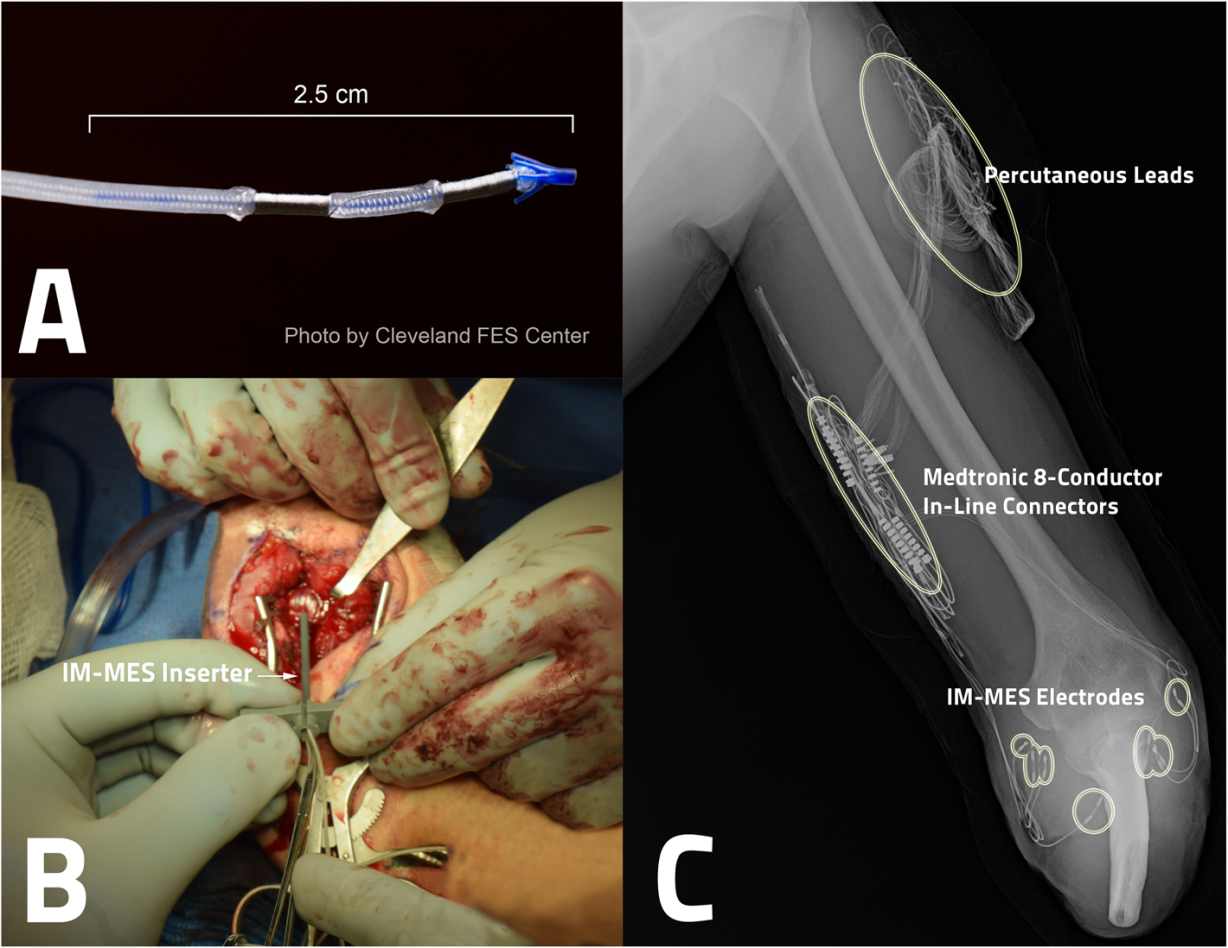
IM-MES Implantation. a One of the eight IM-MES electrodes. b One such electrode being surgically tunneled into a target muscle of the study participant’s residual limb using the IM-MES insertion tool. c X-ray showing the IM-MES electrodes, connectors, and leads following the successful procedure, as well as additional nerve cuff electrodes (unmarked) implanted in the same surgical procedure for use in a separate study
